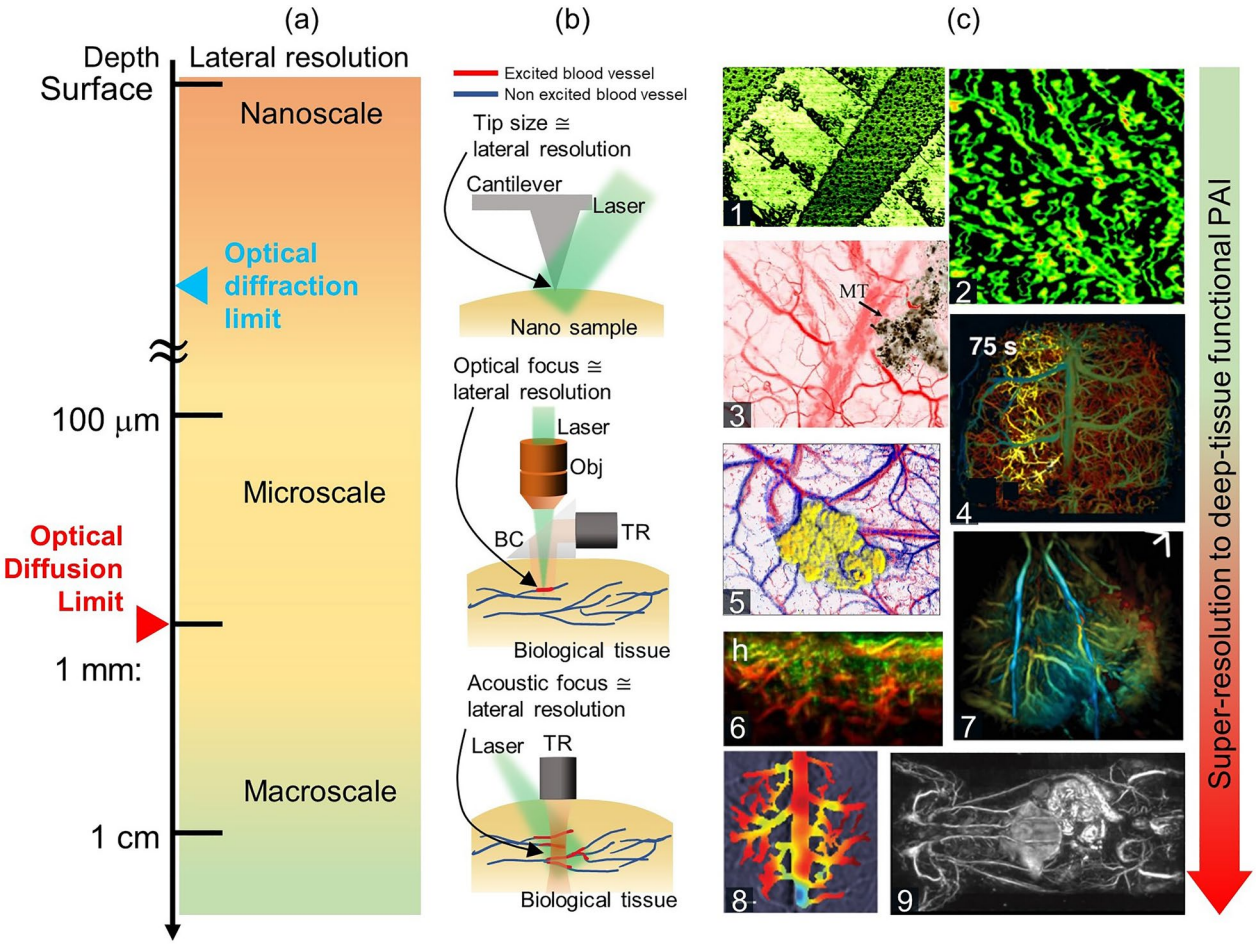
Characteristics of PAI, from surface to cm depth. a PAI depths, from the surface to one cm deep, with corresponding lateral resolutions. b Configuration of PAI for different scales. At nanoscale, microscale, and macroscale, the lateral resolutions are approximated by the sizes of the tip, optical focus, and acoustic focus, respectively. c Representitve super-resolution to deep-tissue functional PA images. Panels 1–9 respectively show an organic semiconductor crack, mitochondria, microvasculature with melanoma, brain vasculature and SO_2_ change, a blood vessel SO_2_ map with melanoma, human melanoma vasculature, the hemodynamics of a fatty liver, the hemodynamics of cerebral, and whole body vasculature, PAI, photoacoustic imaging; PA, photoacoustic; SO_2_, oxygen saturation; TR, ultrasound transducer; BC, beam combiner; Obj, objective lens. The images are reproduced with permission from [17, 24, 25, 82–87]
